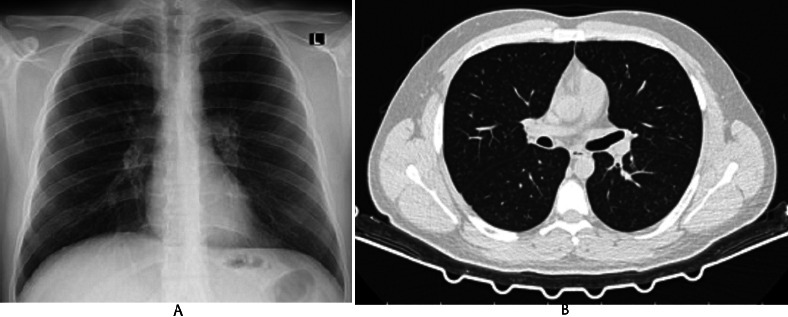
A: ILO pneumoconiosis PA lung X-ray classification p/p 1/1. B: Lung CT with small opacities.
